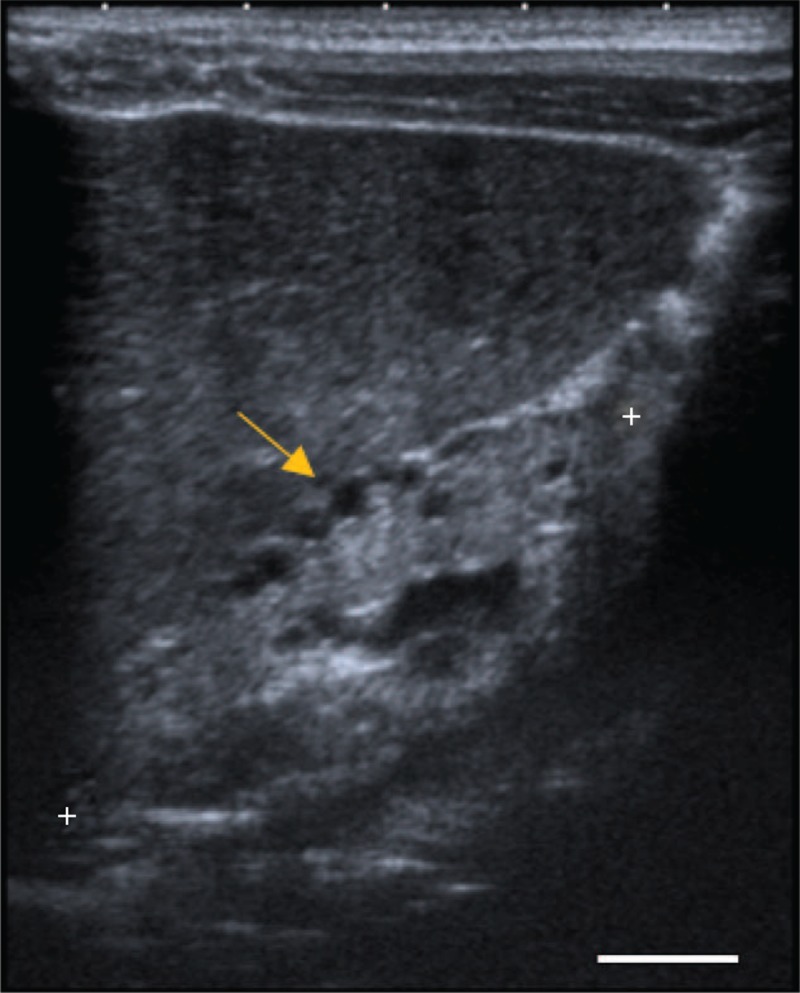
Longitudinal image of renal ultrasound scan performed at 12 months of age. The image shows a small-sized hyperechoic kidney, loss of corticomedullary differentiation, and multiple small cysts with a predominantly subcortical distribution (arrow), a feature that is highly suggestive of glomerulocystic renal disease. The kidney poles are represented as (+). A 10 - mm scale is represented by the horizontal bar.
